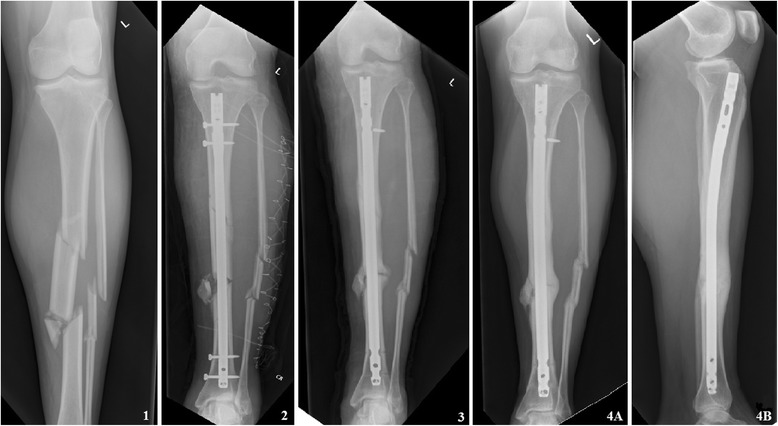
Example of an infection after intramedullary osteosynthesis for a tibial shaft fracture (AO42-C2). 1 At presentation at the emergency department. 2 After nailing, the fracture was accompanied by a compartment syndrome treated with fasciotomy. 3 Patient presented on the emergency department with redness, swelling, and elevated CRP and leukocytes 7 months after initial osteosynthesis. The patient was admitted, and a thorough surgical debridement was performed immediately, the nail was left in situ. Intraoperative tissue cultures were obtained, and empirical antibiotic combination therapy was started. 4 Six months later, the fracture showed bridging of three cortices on X-ray and all signs and symptoms of infection were diminished. The patient visited the outpatient clinic 2 years later for an unrelated issue. The leg was entirely healed without pain with full weight bearing and full range of motion. The nail was never removed
